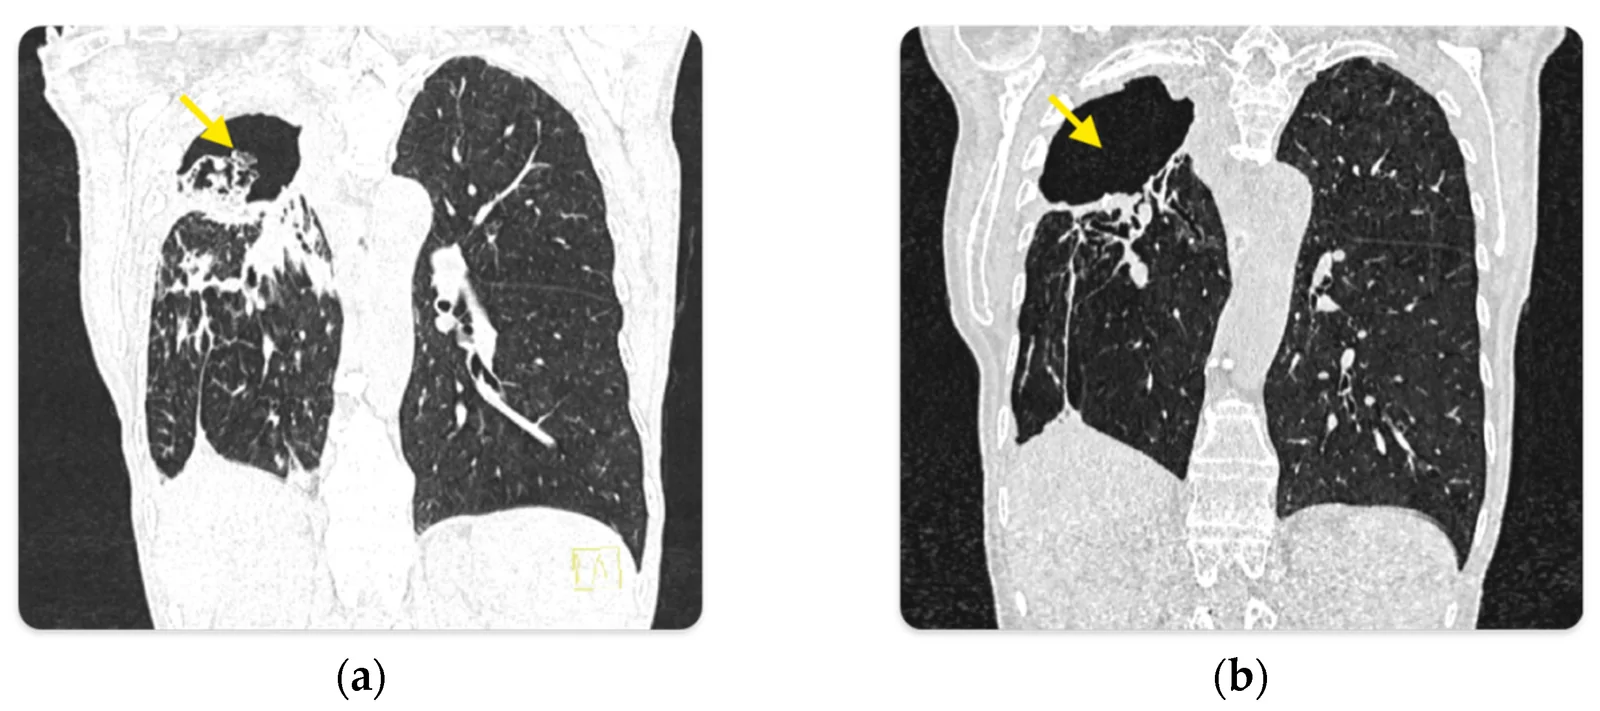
The figure shows the CT scans performed respectively before and after the Isavuconazole treatment: (a) coronal chest CT scan showing extensive air component within the apical right pleural cavity, with an irregular serpiginous structure inside typical of chronic cavitary pulmonary aspergillosis (see yellow arrow in the image); (b) chest HRTC after six months of treatment showing the absence of endocavitary neoformation at the upper right lobe and the almost complete resolution of the underlying consolidation (see yellow arrow in the image).
